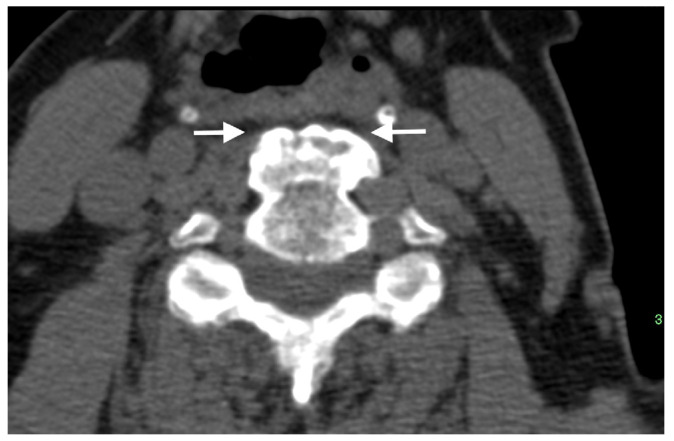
Axial CT image of a thick anterior osteophyte in the cervical spine of a patient with DISH that is impinging and causing narrowing of the adjacent esophagus (arrows).
